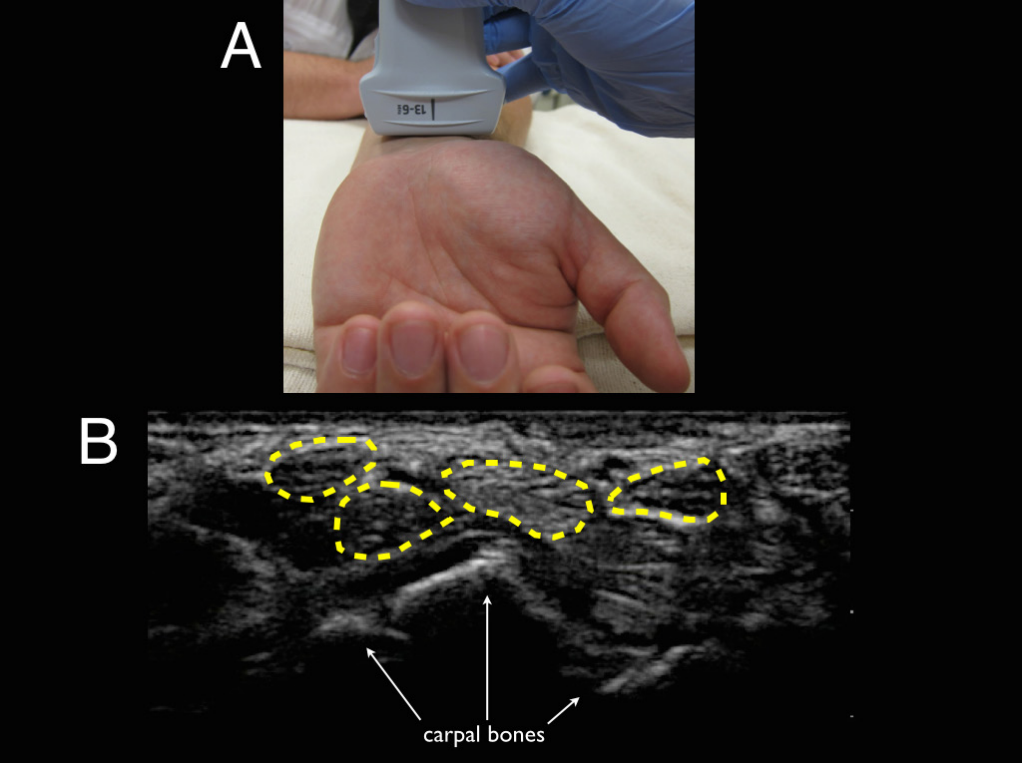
Ultrasound technique and visualization. (A) Appropriate placement of the linear transducer (13MHz to 6MHz) at the wrist crease in transverse plane to evaluate for flexor tenosynovitis. (B) Ultrasound image in transverse view showing normal flexor tendons (highlighted in yellow) with no surrounding edema. The flexor tendons should lie anterior to the carpal bones identified by arrows for reference.
